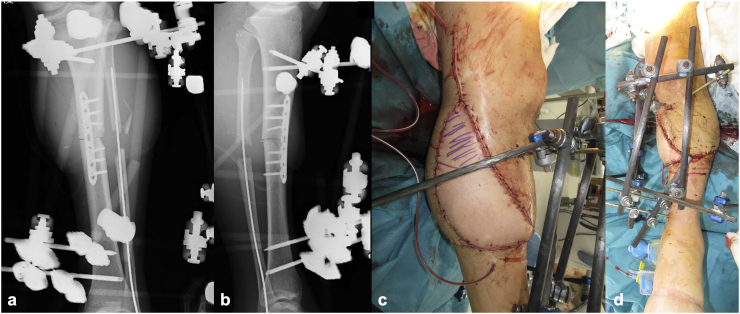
Postoperative radiographic and clinical findings following the ‘Fix and Flap’ technique. (a) Anteroposterior and (b) lateral plain radiographs of the left lower leg after internal fixation. (c) Medial and (d) lateral clinical views showing reconstruction with a free latissimus dorsi musculocutaneous flap.
